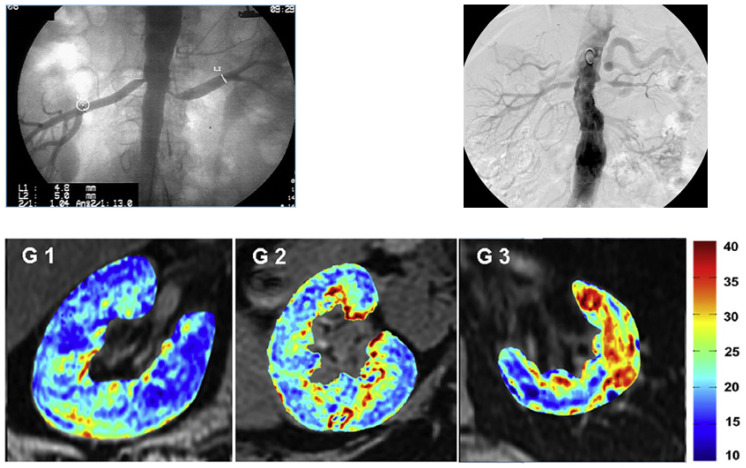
Large vessel atherosclerotic renovascular disease (ARVD) produces reduction in blood flow and loss of kidney volume and glomerular filtration rate (GFR). These changes eventually induce widening tissue hypoxia. The upper panel depicts angiograms with moderate stenoses (left) and more severe occlusion (right) associated with reduced kidney volume and declining glomerular filtration rate (GFR). The bottom panel images depict the increasing intensity of tissue deoxygenation as measured by blood-oxygen-level-dependent (BOLD) MRI in post-stenotic kidneys associated with decreasing GFR. G1: the highest quartile of 48 subjects, GFR: 51 ± 12; G2: middle two quartiles, GFR: 25 ± 6 mL/min; G3: the lowest quartile, GFR: 8 ± 3 mL/min. Data from [4] with permission. Such changes characterize ischemic nephropathy and are associated with progressive inflammation and tissue damage (see text).
